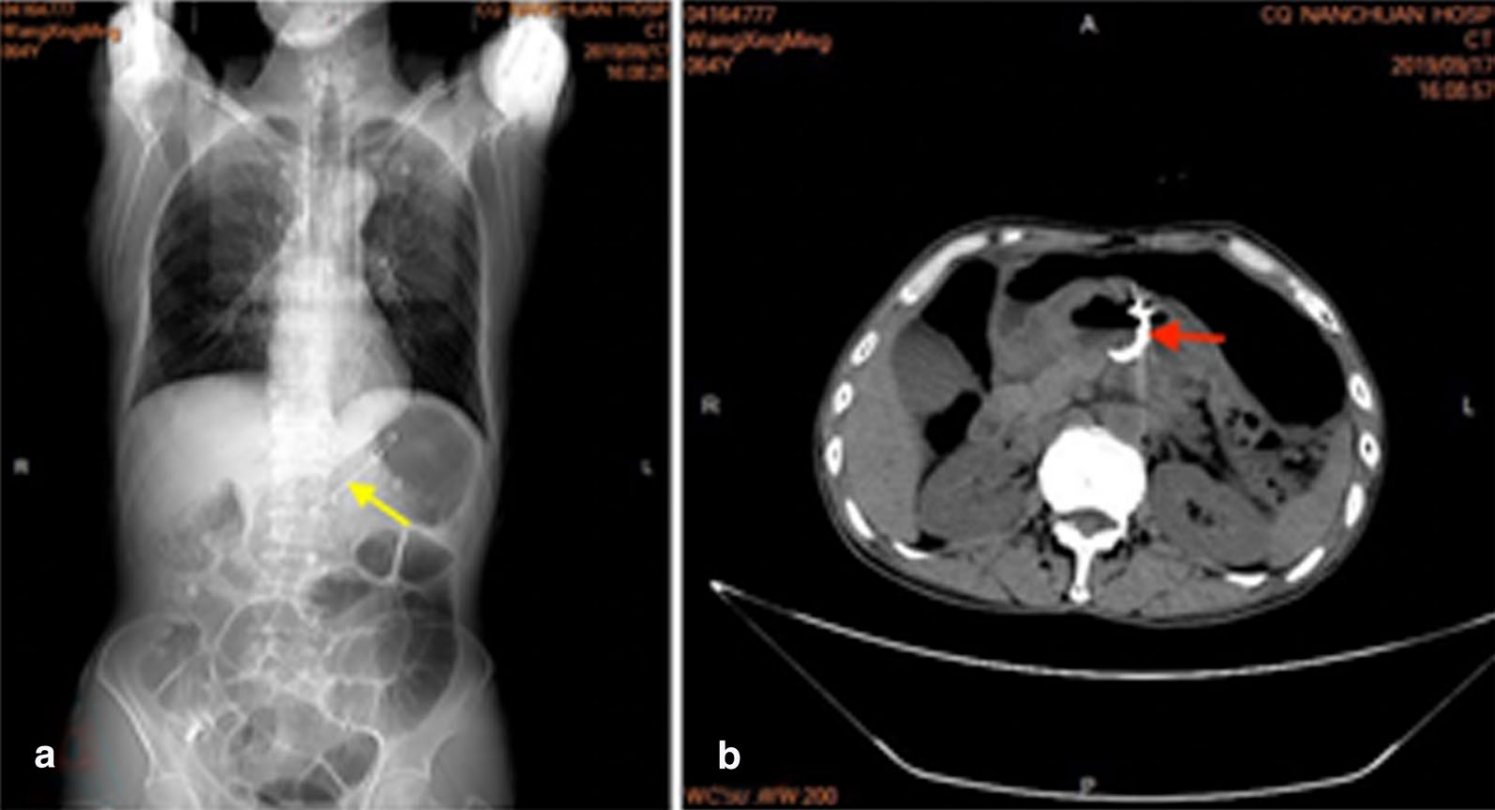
A plastic stent was placed for drainage of the pseudocyst through the pancreas and stomach. a The double pigtail plastic stent (yellow arrow) under X-ray. b One tail of the stent (red arrow) is located in the pseudocyst
